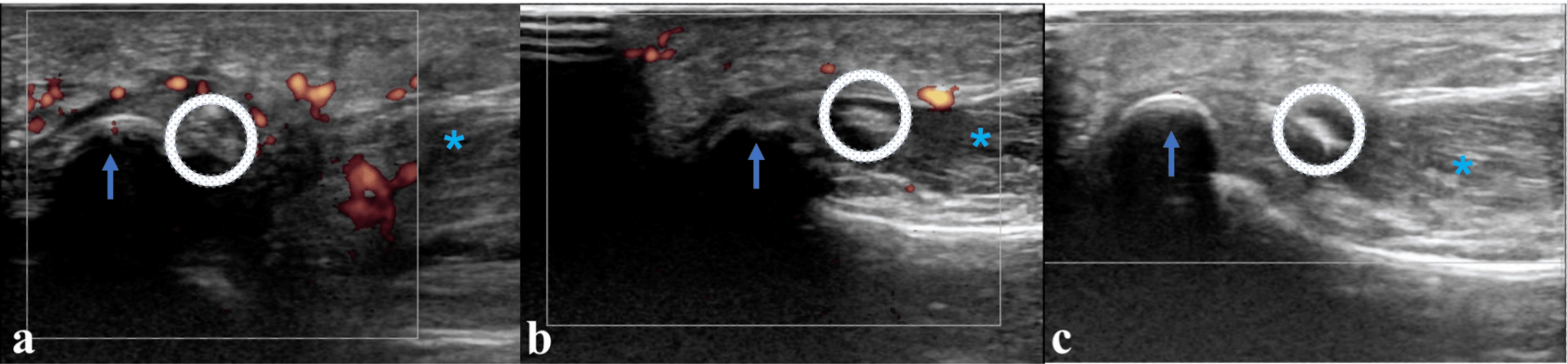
An ultrasound The arrows indicate the sesamoid bone. The asterisks indicate the flexor hallucis brevis. The blue circles indicate calcification. (a) Initial ultrasound: significant inflammation was visible around the calcification in the color Doppler imaging mode of the ultrasound. (b) Secondary follow-up. (c) Final follow-up: inflammatory changes were not observed during the final follow-up.
